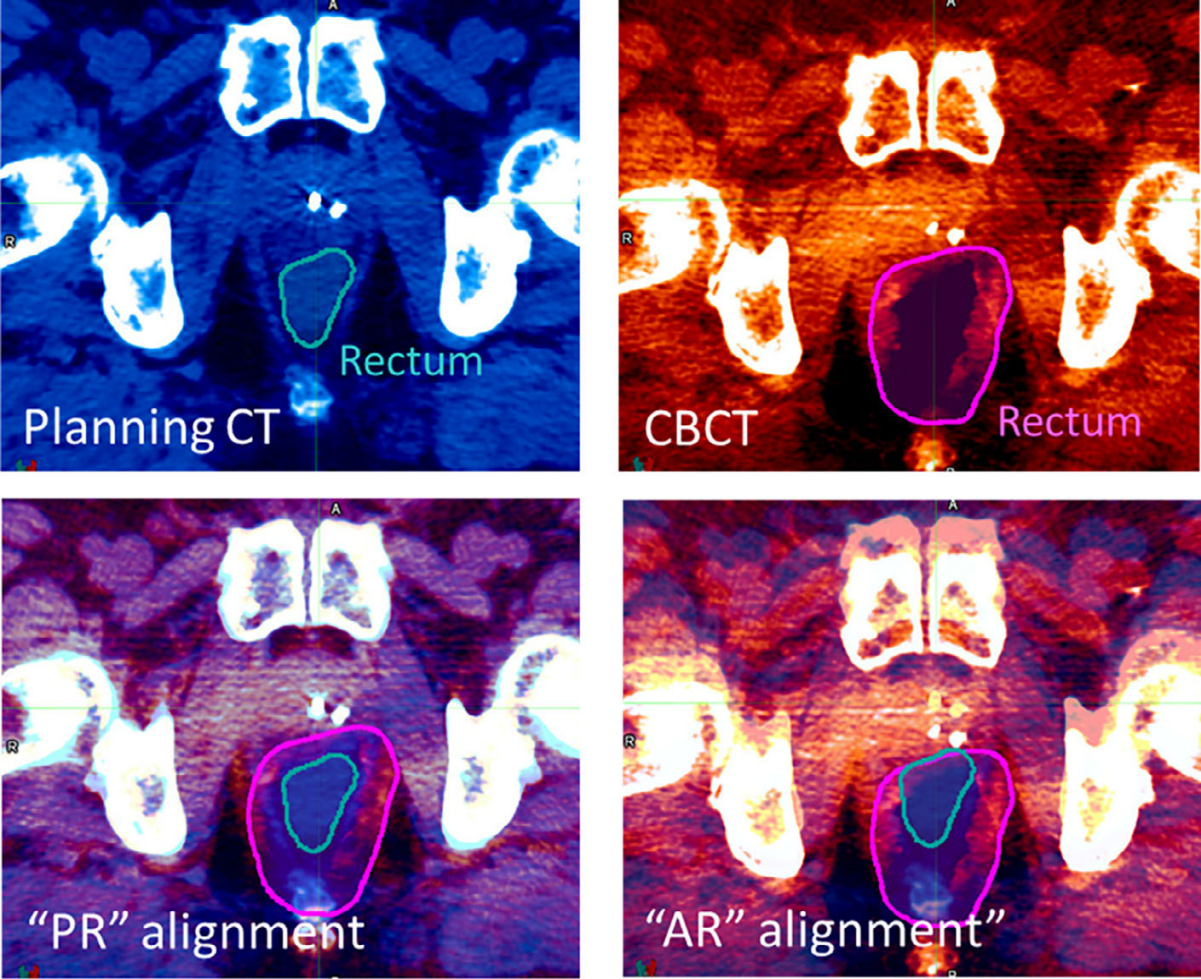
Prostate (“PR”) versus anterior rectum (“AR”) alignment. The rectum is contoured on the planning CT and on the daily CBCT (top row). The “PR” alignment is executed by aligning the fiducial markers inside the prostate. The “AR” alignment aims to align the anterior wall of the rectum. Note no perfect alignment is possible for either “PR” or “AR”, due to the non‐rigid nature of the involved anatomy. CBCT, cone beam computed tomography.
